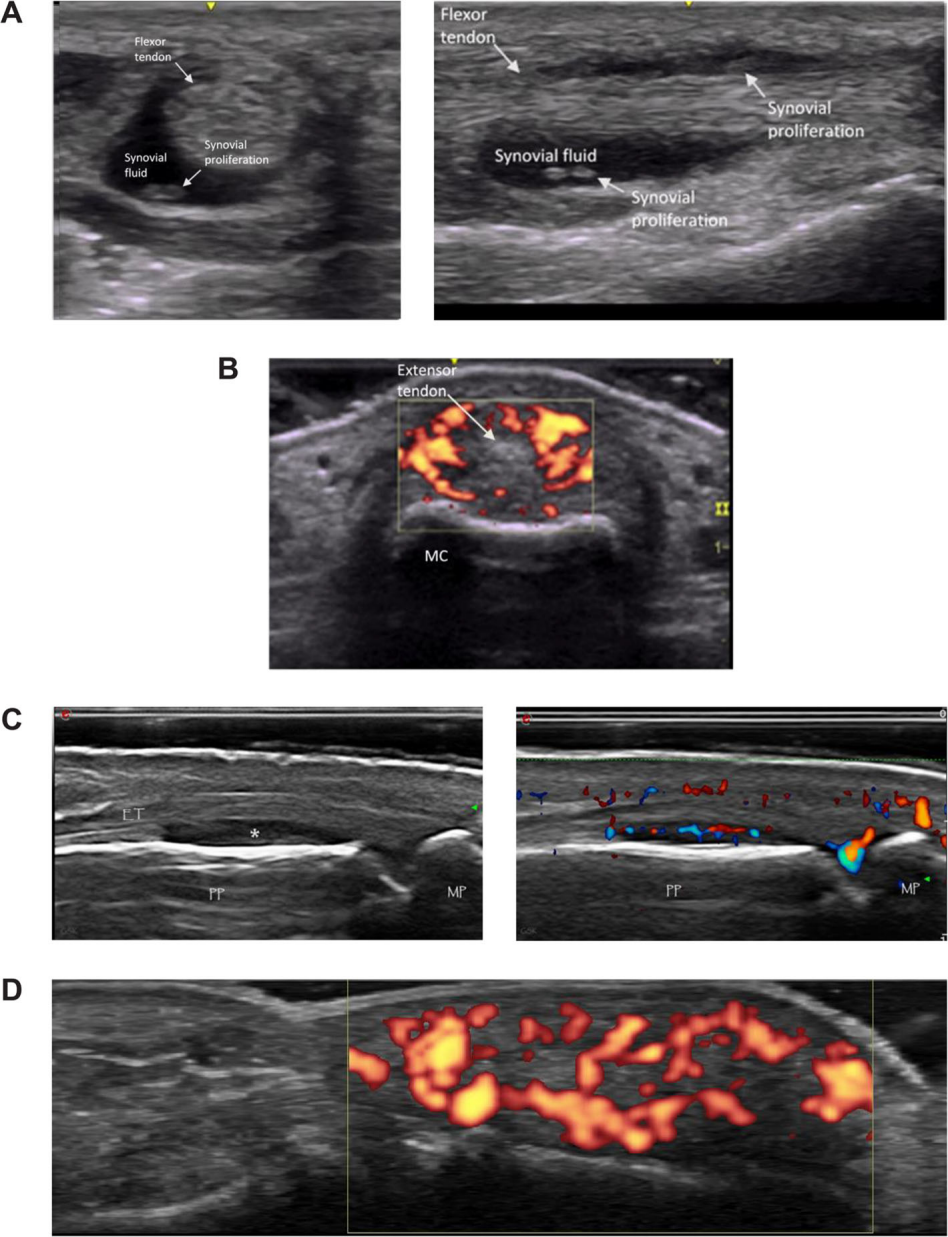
Ultrasound imaging of synovitis and tenosynovitis. a Flexor tenosynovitis in transverse (left) and longitudinal (right) views. b Metacarpophalangeal joint paratenonitis, dorsal aspect of second metacarpophalangeal joint. MC, metacarpal. c Dorsal proximal interphalangeal B-mode (left) and power Doppler (right) images indicating synovitis in the recess (asterisk). PP, proximal phalanx; MP, middle phalanx; ET, extensor digitorum tendon. d Positive power Doppler signal of finger pulp
